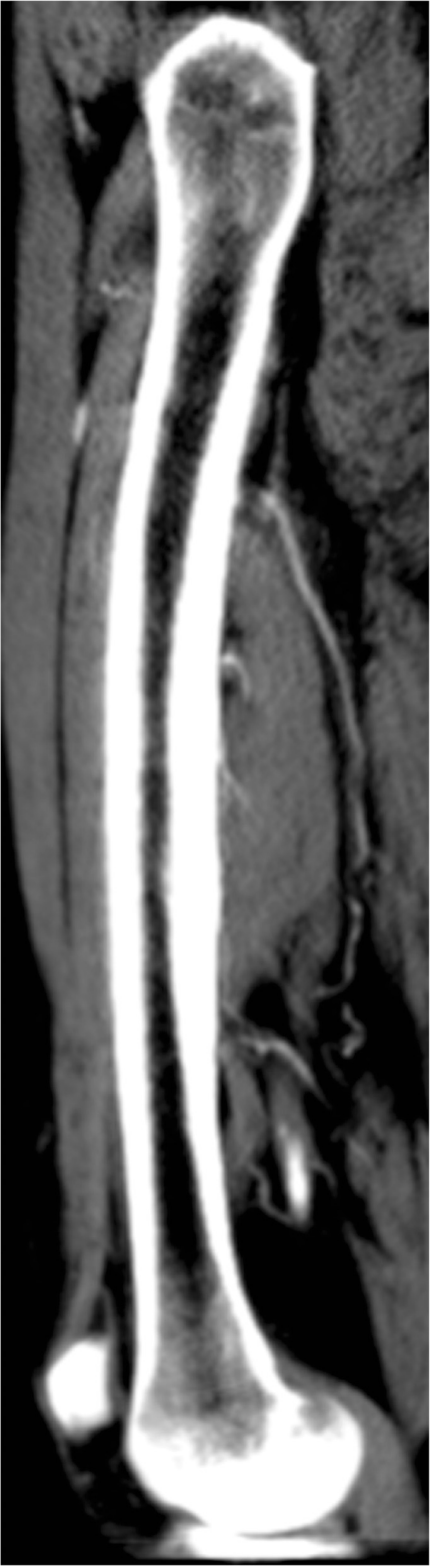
Computed tomography image of the femur in the sagittal section; the medullary canal has a greater antecurvature than that of the whole femur
